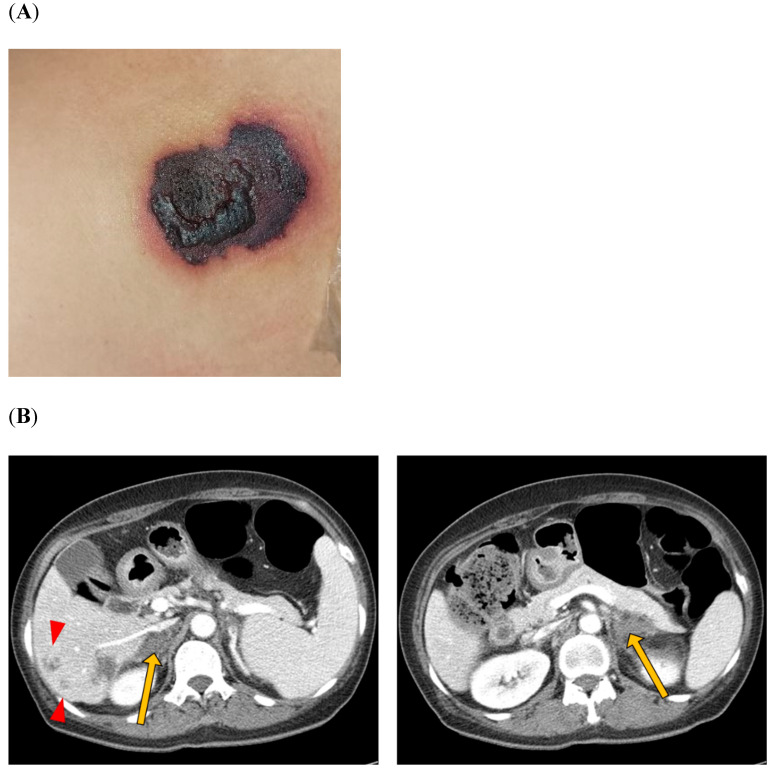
(A) Hemorrhagic bullae with erythema located on the abdomen. (B) An abdominal computed tomography scan showing multiple low attenuating lesions in the bilateral adrenal glands (yellow arrows) and low attenuating lesions in the liver (red arrowheads).
